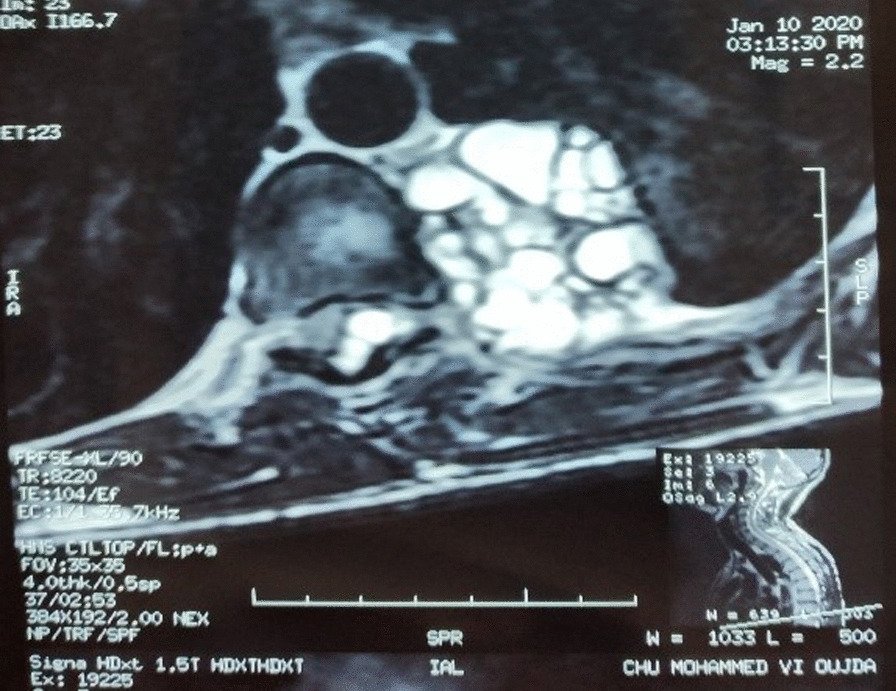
MRI showing costovertebral hydatid cyst with the presence of endocanal vesicles suggesting an invasion of the bone marrow with risk of medullary compression
